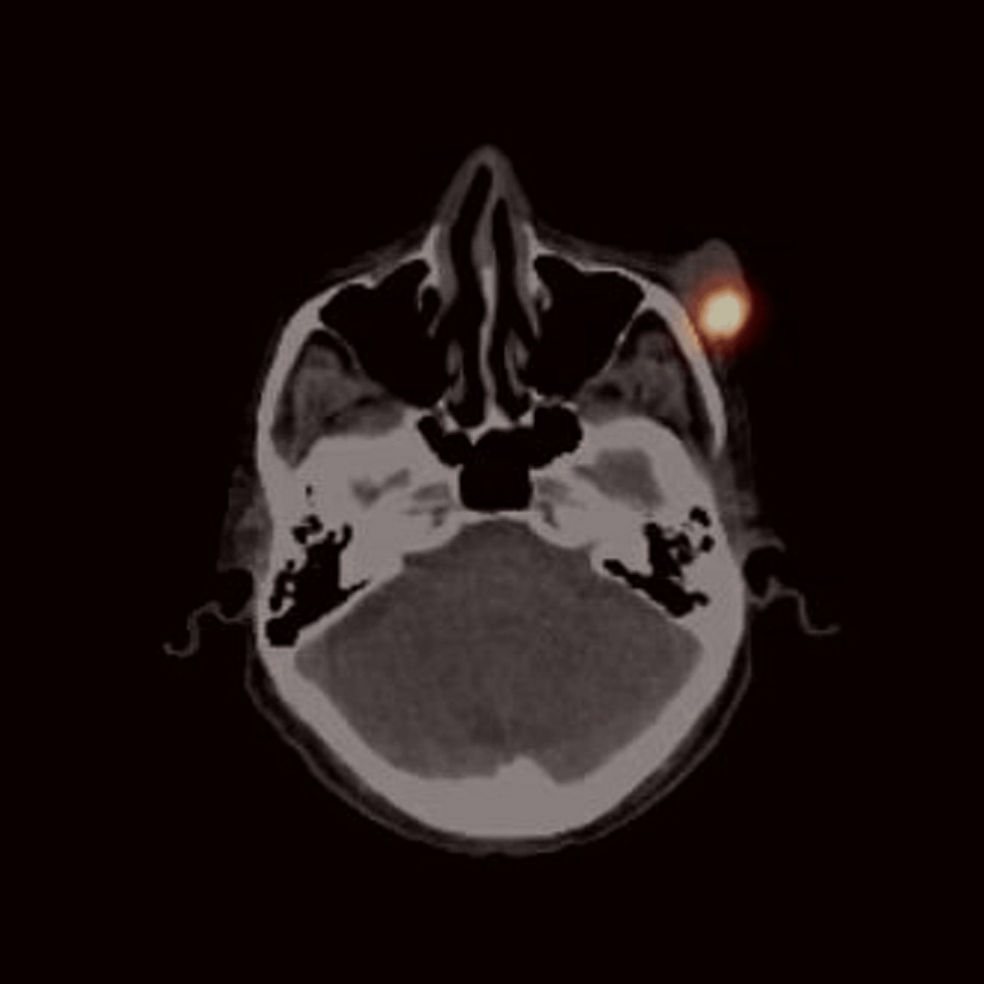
Axial image of fused/SPECT with intralesional injection of Tc99. There were no radiographic foci outside of the lesion that would indicate a sentinel lymph node. SPECT: single-photon emission computerized tomography; Tc99: technetium-99
